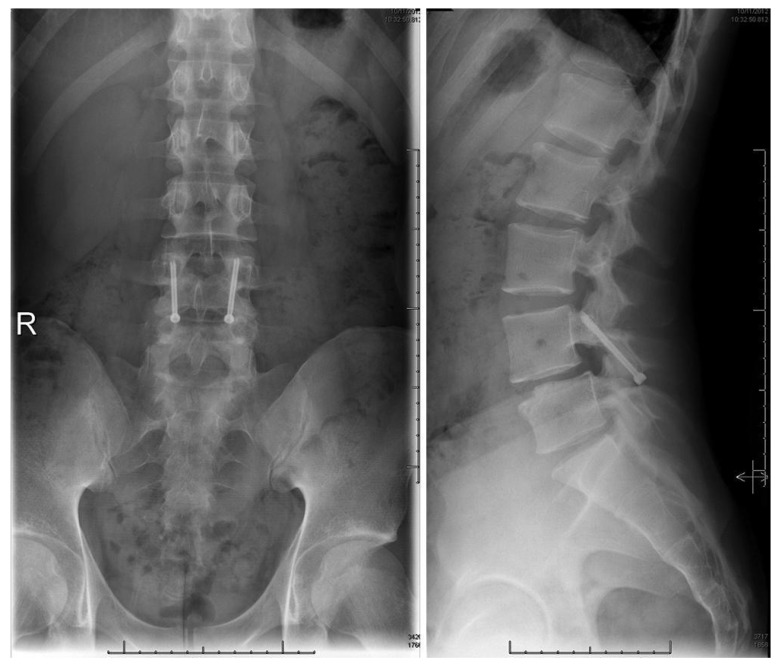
Postoperative upright standing radiographs in AP (left) and lateral (right) projections demonstrating bilateral placement of lag screws [68]. “Reproduced with permission from Ghobrial, G.M.; Crandall, K.M.; Lau, A.; Williams, S.K.; Levi, A.D. Minimally invasive direct pars repair with cannulated screws and recombinant human bone morphogenetic protein: case series and review of the literature; published by Neurosurg. *Focus* 2017, *43*, E6”.
